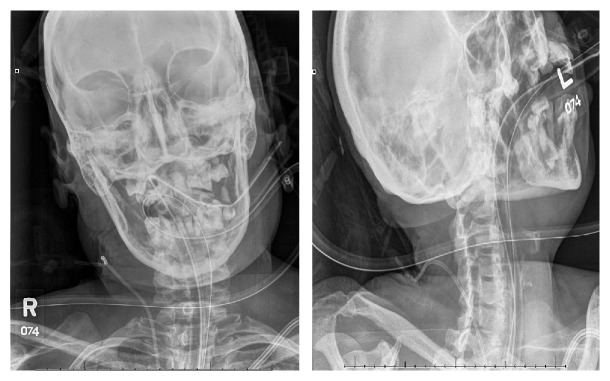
X-ray of the skull, showing lytic lesions in the mandible and bilateral maxillae.
